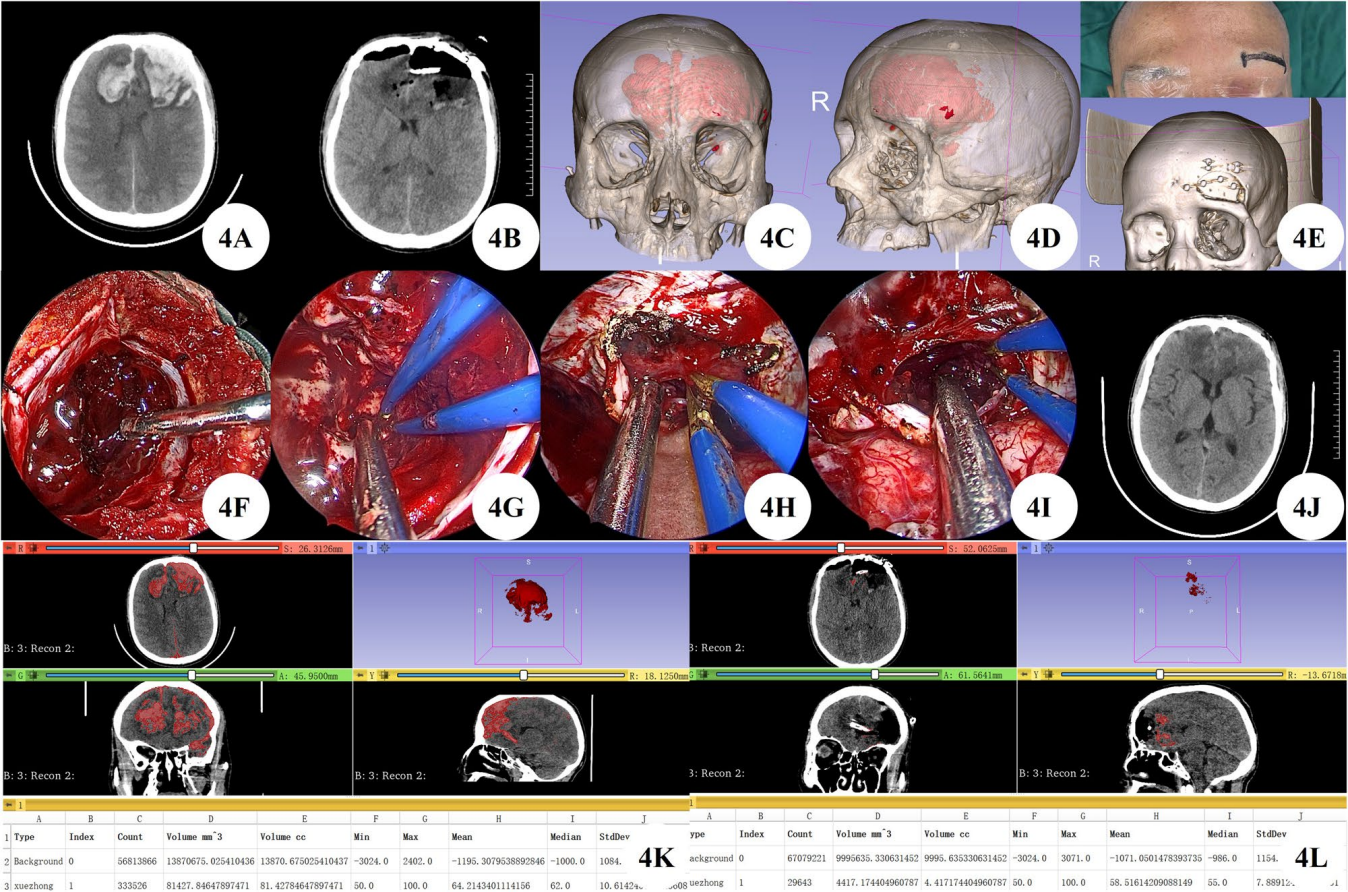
(A) Preoperative CT shows bilateral frontal lobe contusion and hematoma formation. (B) Postoperative CT shows bilateral frontal lobe brain contusions and hematoma cleared completely. (C,D) 3D Slicer reconstruction of virtual reality images of hematoma for preoperative evaluation. E: Surgical incision and bone window. (F,G) Clearing ipsilateral brain contusion and hematoma by neuroendoscopy. (H,I) Neuroendoscopic incision of cerebral falx and removal of contralateral brain contusion and hematoma. (J) 20 days post-surgery, CT scan showed good recovery. (K–L) 3D Slicer accurately calculates preoperative and postoperative hematoma volume.
